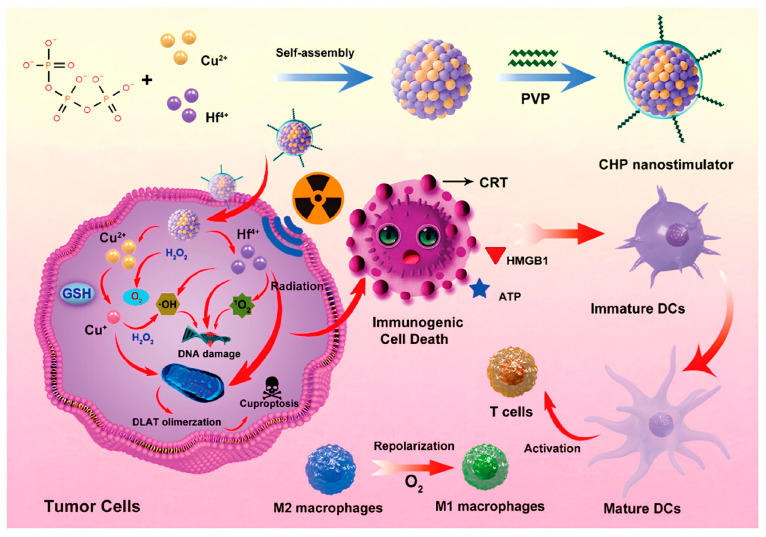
The bimetallic hybrid nanoregulator constructed through phosphate backbone coordination of Cu^2+^ and Hf^4+^ can be specifically activated within the acidic tumor microenvironment. The subsequently released Hf^4+^ interact physically with X-rays, triggering electron release and generating ROS, ultimately leading to irreparable double-strand DNA breaks and tumor cell apoptosis. Simultaneously released Cu induces cascaded amplification of oxidative stress by depleting GSH while effectively triggering cuproptosis. These synergistic mechanisms collectively enhance ICD, thereby successfully activating a systemic antitumor immune response under low-dose radiotherapy conditions. Reproduced with permission from [143].
